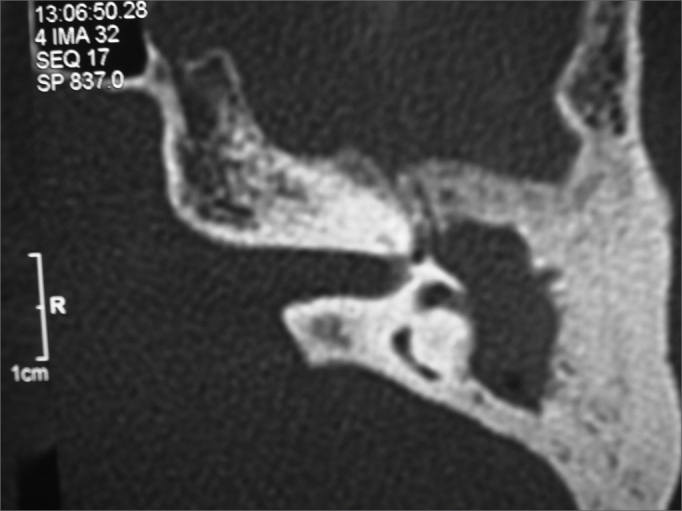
Left-side temporal bone CT scan, axial cross-section, showing blurred antrum and erosion of the lateral semicircular canal.
